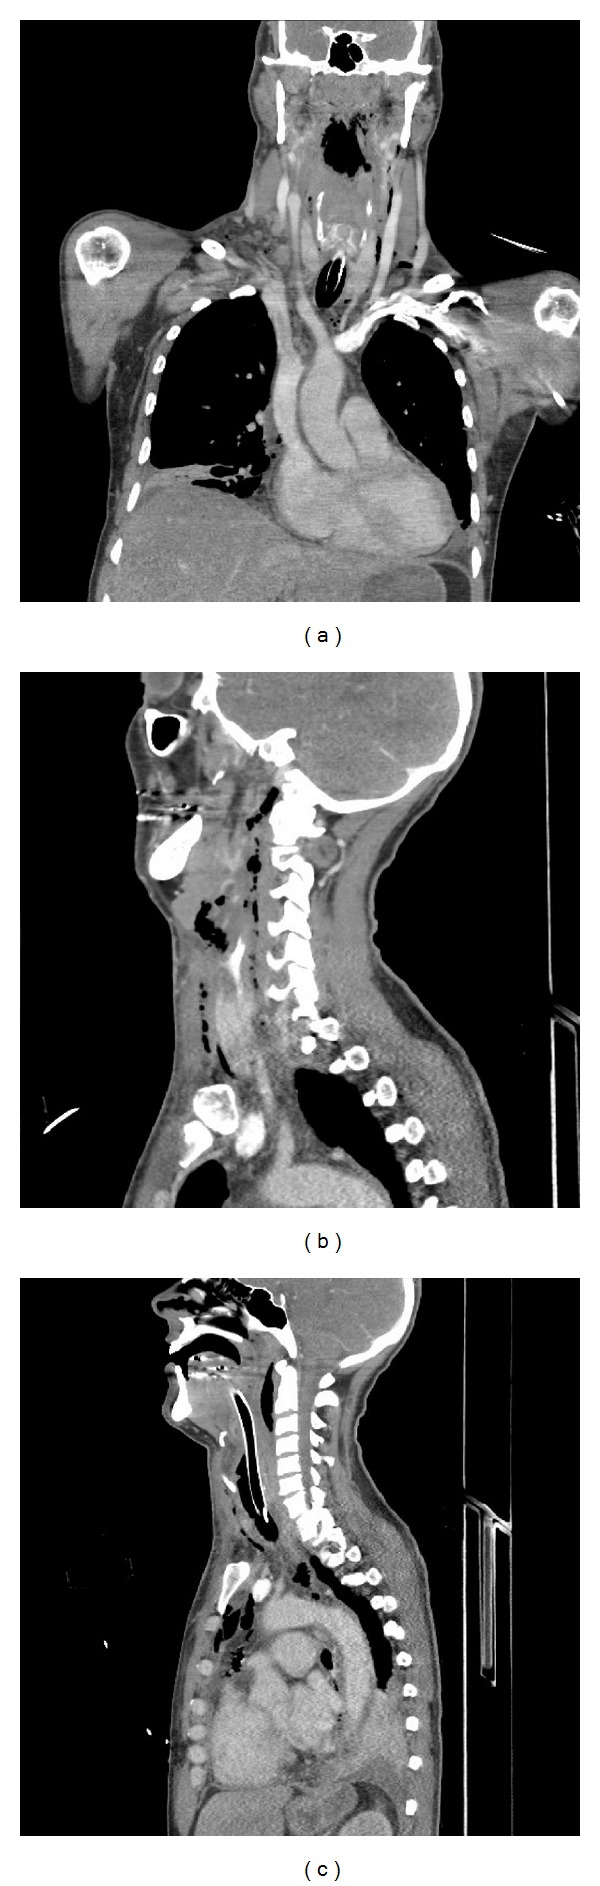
Coronal and sagittal CT scans demonstrating free gas from the skull base through the neck and into the anterior and posterior mediastinum.
